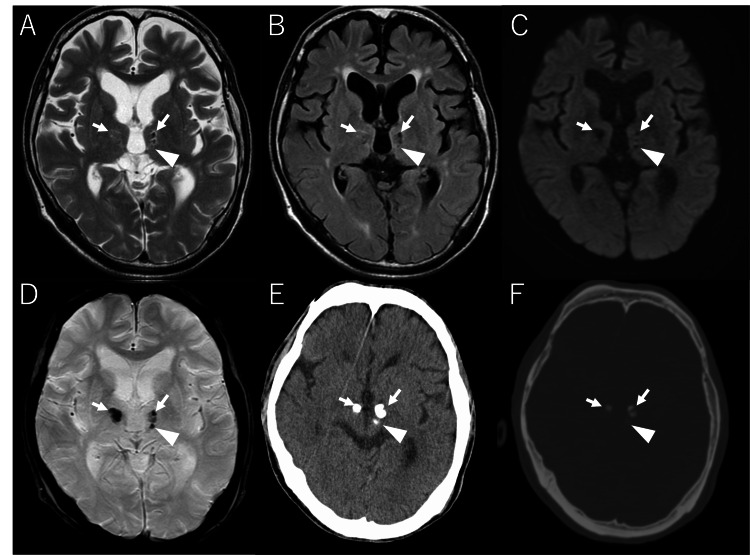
Current MR images (A-D); CT images from five years ago (E-F). Lesions are observed in the bilateral thalami (arrows) and left subthalamic nucleus (triangle). The central areas of the lesions show low signal intensity on T2WI (A), FLAIR (B), DWI (C), and T2*WI (D). On T2WI, high signal intensity is observed at the margins. CT demonstrates obvious hyperdensity (E,F). FLAIR: fluid-attenuated inversion recovery, DWI: diffusion-weighted imaging.
